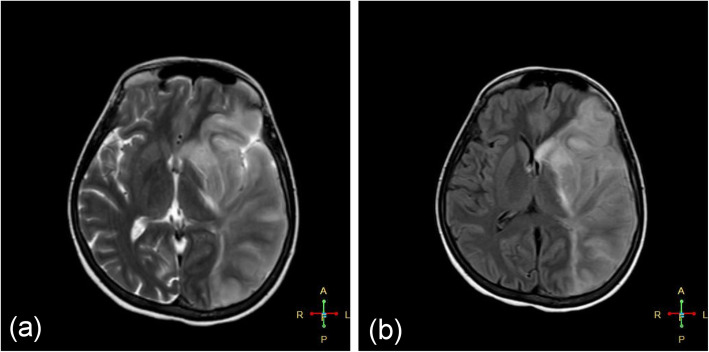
Axial MRI of the patient’s brain revealing a large hyper-intense area in the left parietal lobe corresponding to left middle cerebral artery (MCA) distribution (a; T2-weighted sequence, b; T2- weighted fluid-attenuated inversion recovery (FLAIR) sequence)
